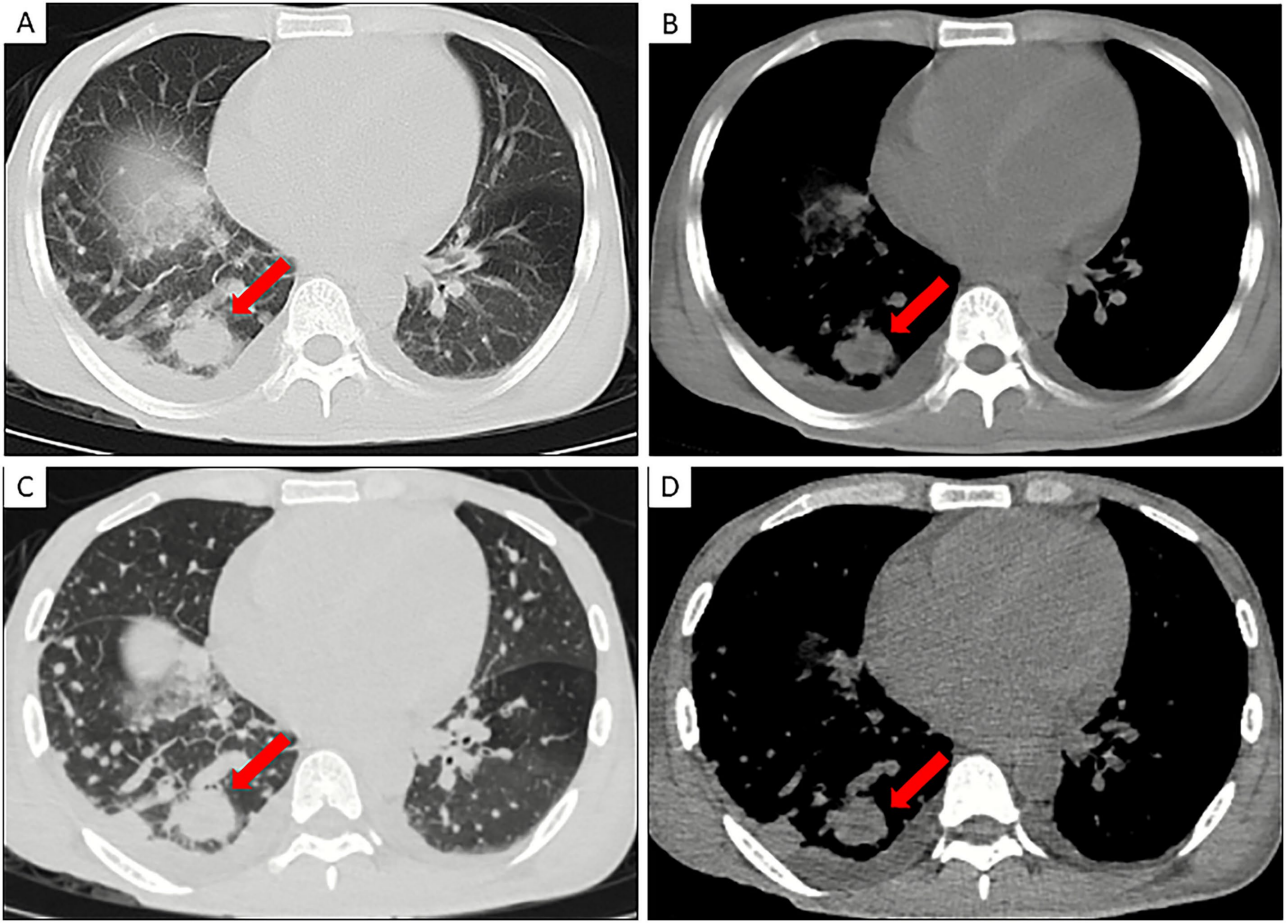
A 29-year-old male, suffering from Mpox pneumonia, initially presented with fever and a history of risky homosexual behavior. (A,B) CT scans in lung window (A) and mediastinal window (B) reveal a solid nodular lesion in the posterior basal segment of the right lower lobe (red arrow), measuring approximately 36 mm in diameter (considering its origin, it is still classified as a nodule rather than a mass). (C,D) thin-section reconstructions in lung window (C) and mediastinal window (D) show the lesion with irregular borders, characterized by conspicuous spiculation and lobulation.
